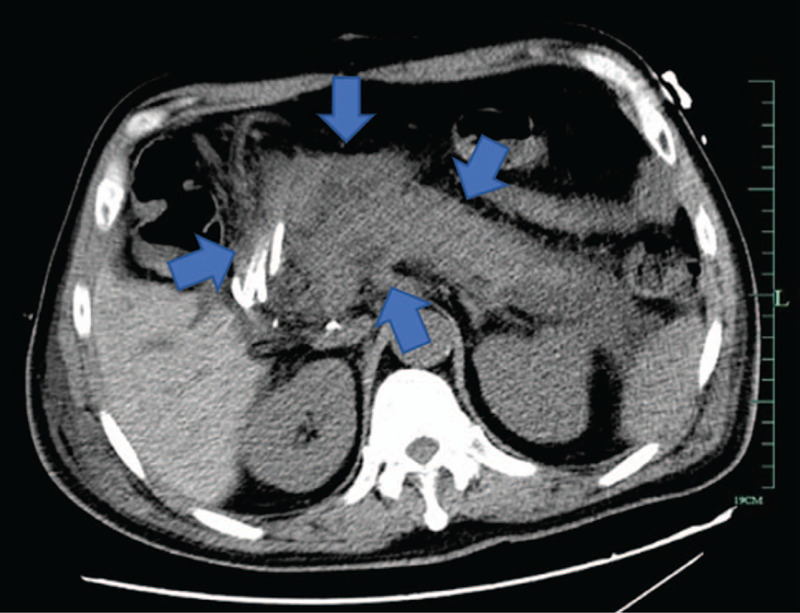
After surgery, abdominal CT showed pancreatic exudation.
